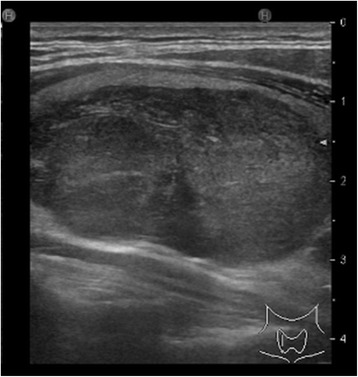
Ultrasound showed a solid hypoechoic nodule with taller than wide shape in the right robe of the thyroid gland
